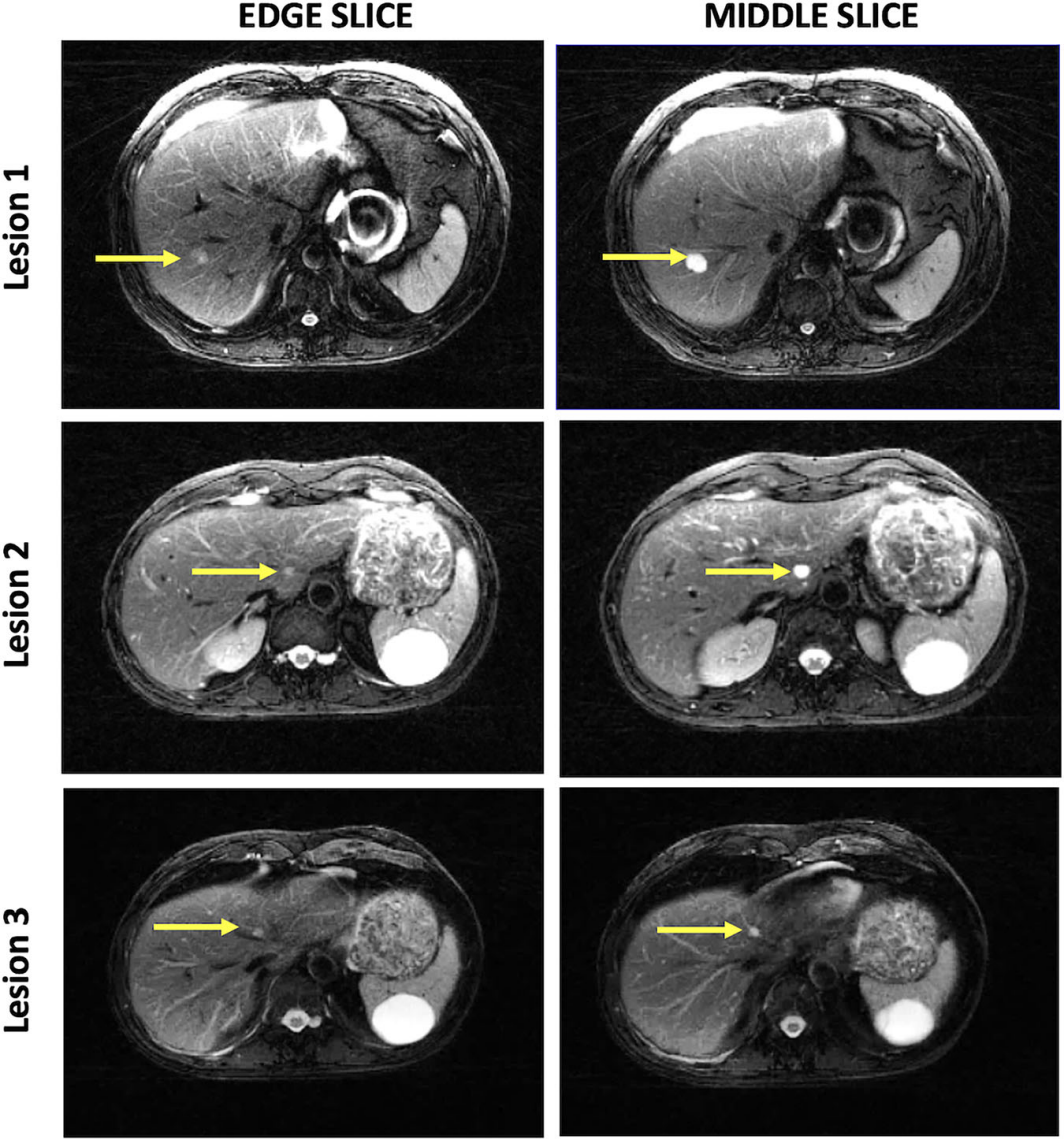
T2-weighted images of three lesions showing the slices at the edge and through the middle of these lesions. The T2 values of ROIs corresponding to the edge slices are shown in Fig. 3 (asterisks)
